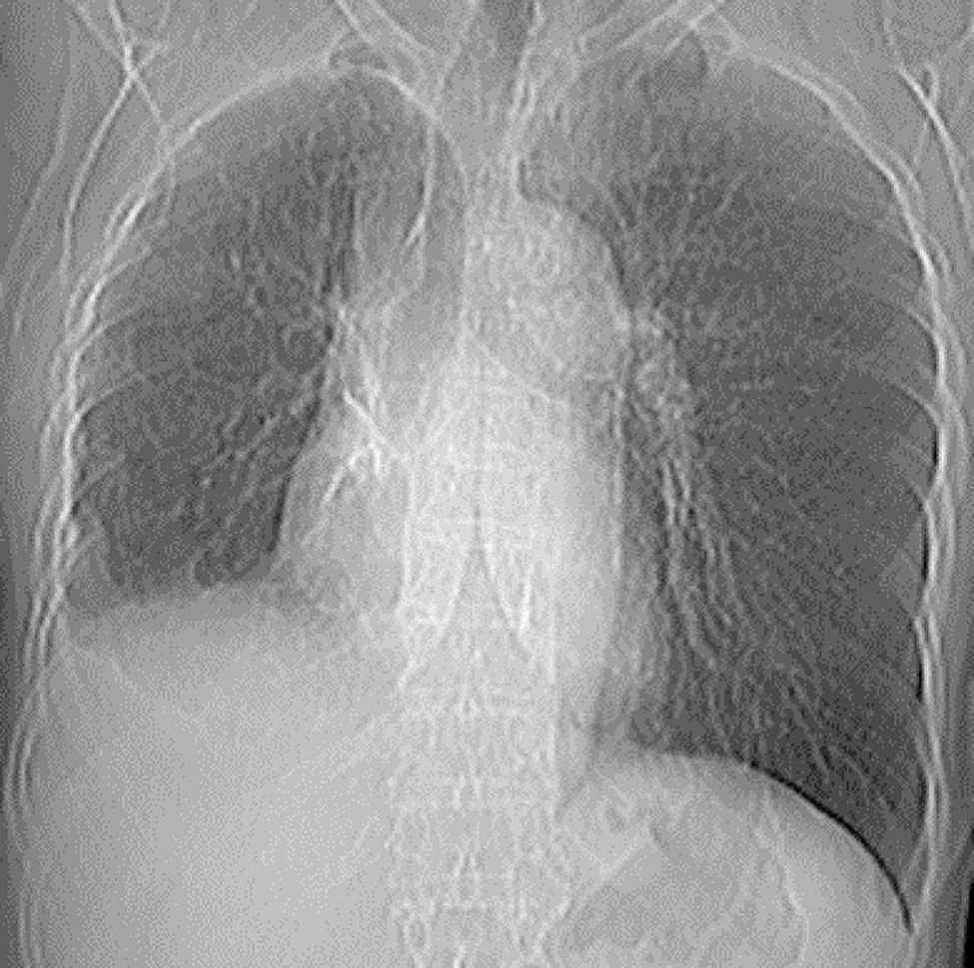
Her follow-up chest x-ray after 6 months of the third operation
